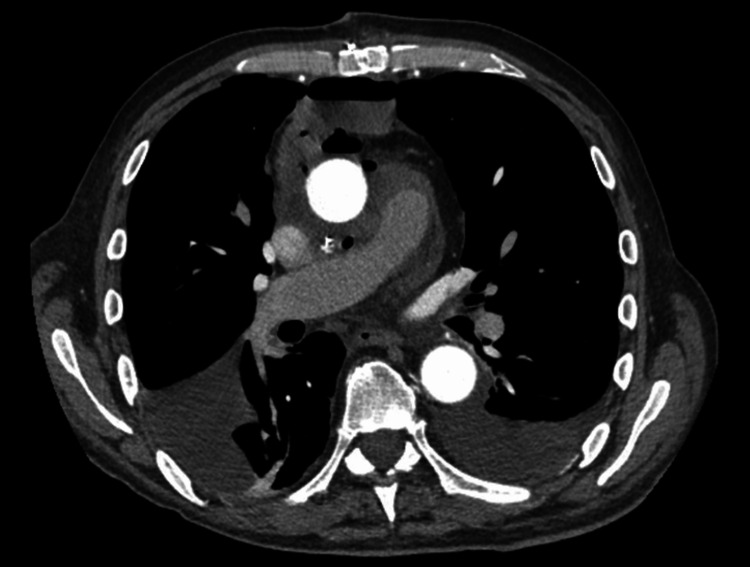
CT aorta 10 days after repair Normal-caliber thoracic aorta with no evidence of extravasation and a significant decrease in the size of the hematoma.
